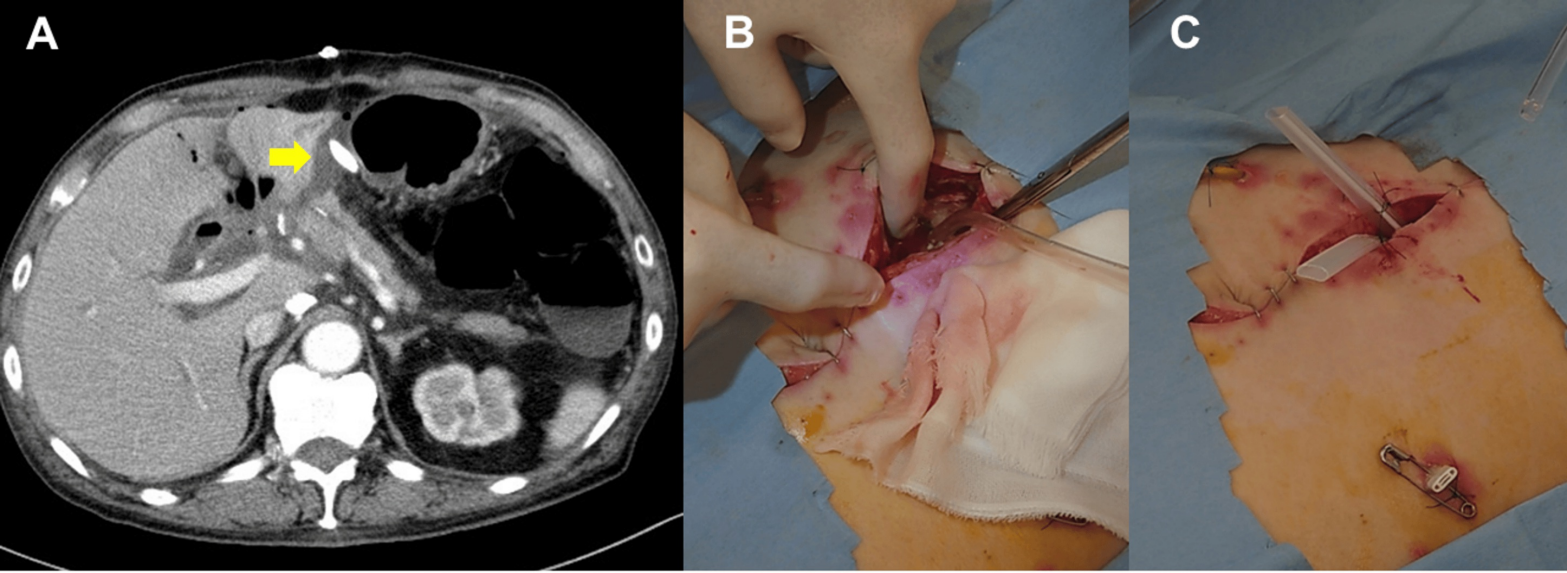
The open drainage procedure. A. A contrast-enhanced computed tomography image shows fluid collection between the lateral section of the liver and pancreatojejunostomy (arrow) despite a catheter drain, which was intraoperatively placed. B. After removing the fascial sutures, the fluid collection is opened manually under direct vision. C. A view after open drainage. A Penrose drain is placed to maintain the drainage route. The drain at the left side of the abdomen will be exchanged under fluoroscopy later.
